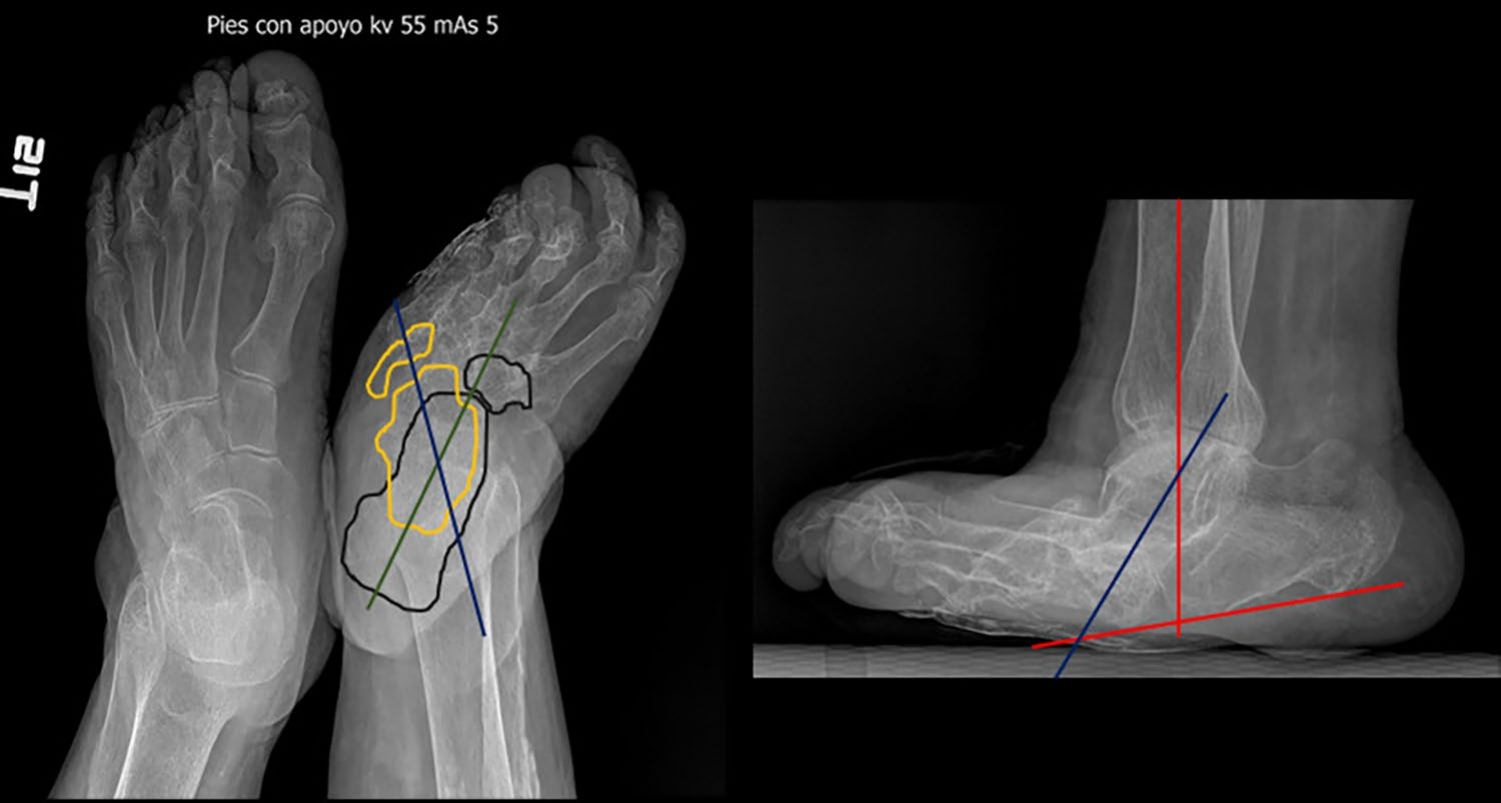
Comparative plain foot radiographs, anteroposterior projection and right foot radiograph with lateral projection support, of an 86-year-old patient with complex regional pain in the left foot associated with chronic osteomyelitis of the talus, tibial malleolus and distal metaphysis of the right tibia since 2016 with amputation of the 1-2 artery in 2017. Findings of the right foot: Changes due to chronic hypertrophic neuropathic arthropathy in the coalescence or remodelling phase. Absence of the phalanges and metatarsals of the first toe. A: Calcaneal deformity of the hindfoot in front of a tibiocalcaneal angle of less than 60° (see-saw deformity). Talonavicular subluxation (bones marked in yellow and joint axis marked in blue). Cuboidal calcaneal subluxation (bones outlined in black and axis outlined in green). Varus deformity of the 3rd and 4th metatarsophalangeal joints. B: Diffuse decreased bone density, sclerosis and subchondral cysts and marginal osteophytes of the forefoot, midfoot (predominantly) and hindfoot joints. C: Ankylosis of the wedge-metatarsal and cuboid-calcaneal joints. D: Mainly distributed over the midfoot and hindfoot. E: No erosions. S: Oedema of the superficial soft tissues.
